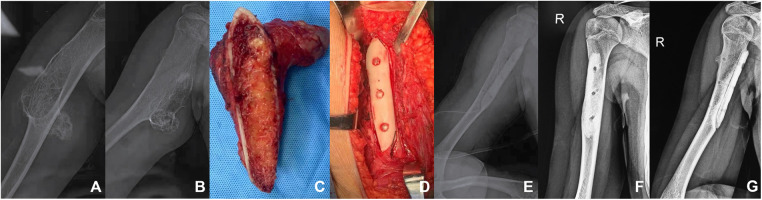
A patient with humerus osteochondroma. (A,B) The preoperative plain radiographs of the patient showed a huge osteochondroma at the proximal end of the right humerus, with a size of 12 cm * 5 cm * 5 cm. (C) Osteotomy was performed from the base of the tumor. (D) The allograft was implanted in the bone cortical defect and fixed with three absorbable screws. (E) Immediate postoperative radiographs. (E,F) Six months after the operation, the plain radiographs of the right humerus showed that the effect of bone graft fusion was desirable.
